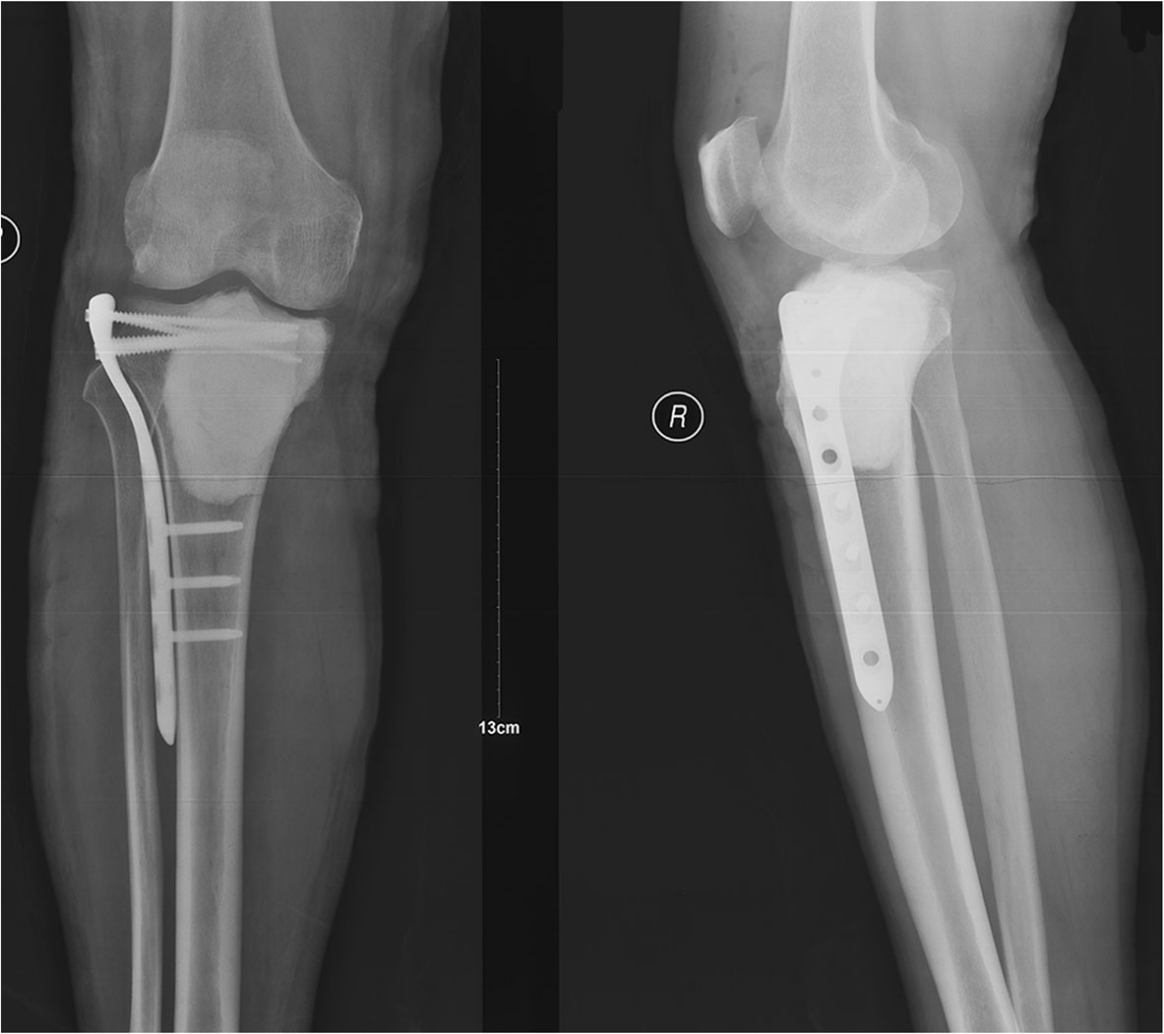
The postoperative X-ray film of the right tibia
